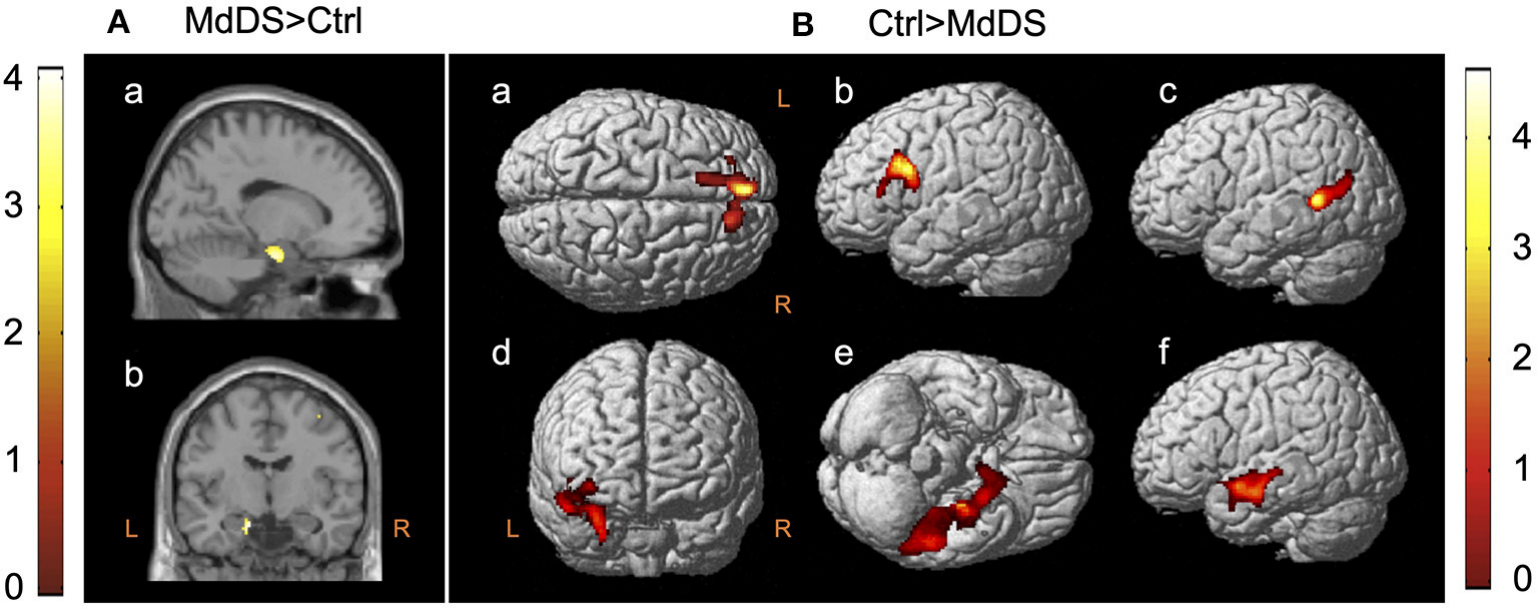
^18^F-FDG PET contrasts between mal de débarquement syndrome (MdDS) and controls (Ctrl) with differences of *z* > 3.3. Clusters are shown at *z* > 2.57 for the MdDS > Ctrl contrast and at *z* > 1.96 for the Ctrl > MdDS contrast for better visualization. Coordinates of peak significance are indicated in parentheses. In SPM, negative *X*-values are on the left and positive on the right. (A) MdDS > Ctrl: left entorhinal cortex/amygdala [Montreal Neurological Institute (MNI): −14, −8, −22], (a) sagittal view and (b) coronal view. (B) Ctrl > MdDS: (a) left superior medial gyrus (MNI: −8, 52, 36), (b) left middle frontal gyrus (MNI: −32, 18, 30), (c) left middle temporal gyrus (MNI: −50, −52, 8), (d) right insula/amygdala (MNI: 30, −2, −26 and 40, 2, −8), (e) left inferior temporal gyrus (MNI: −52, −38, −24), and (f) left superior temporal gyrus (MNI: −52, 0, −14). Figure adapted from Cha YH et al., *PLOS One* 2012 (4).
